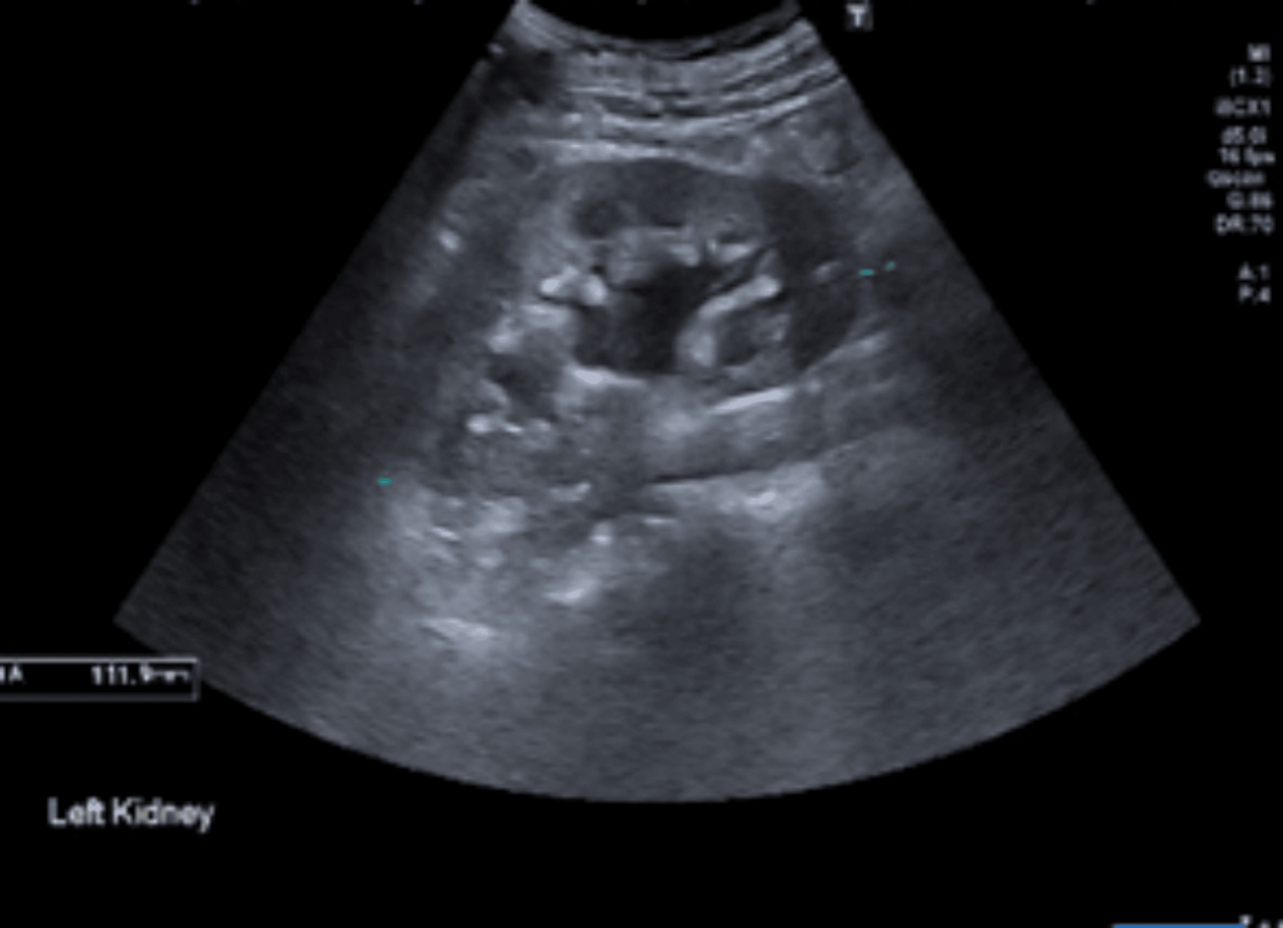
Ultrasound of the left kidney showing hydronephrosis.
